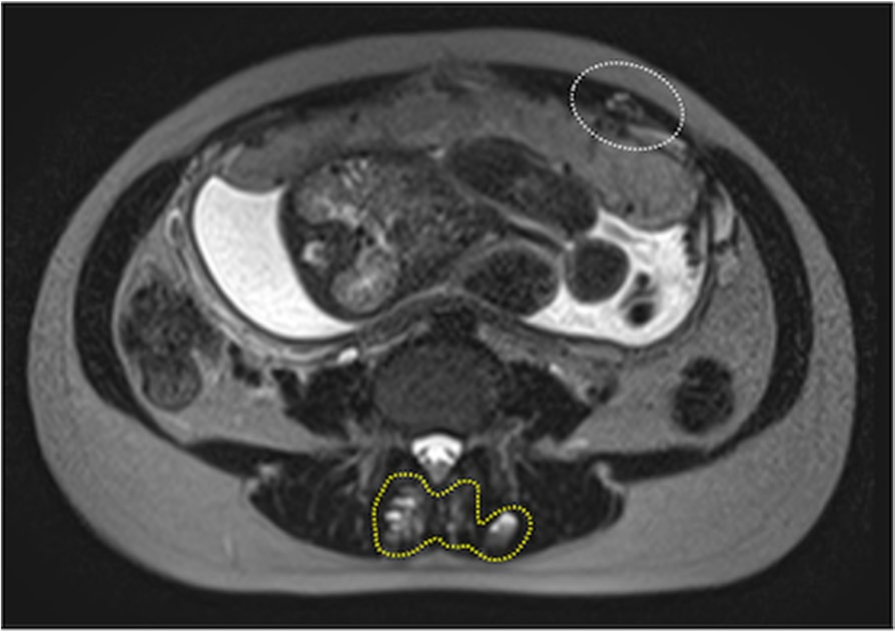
Transverse view of MRI between the third and fourth lumbar vertebrae. The MRI T2-weighted image shows multiple vascular malformations in the lumbar multifidus muscles (circled by the yellow dashed line), and the lateral edge of the left rectus abdominis muscle (circled by the white dashed line)
